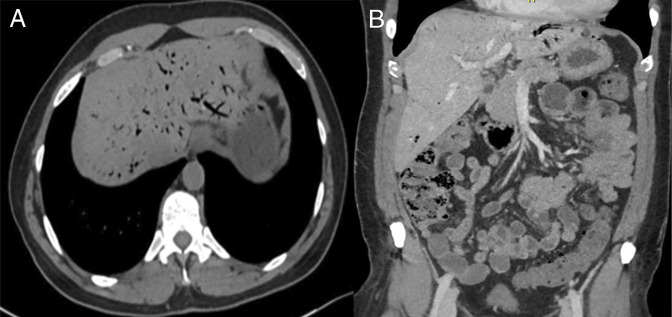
Axial computed tomography image of the abdomen demonstrating extensive portal venous gas in both liver lobes (A). Coronal view showing multiple tubular gas locules in the sigmoid mesocolon, consistent with mesenteric venous gas (B).
